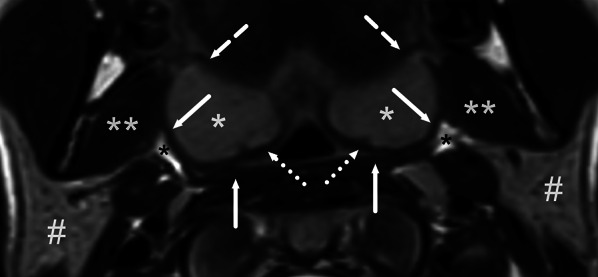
Normal MRI anatomy of the oropharynx. Axial T2-weighted image shows the palatine tonsils (white asterisk) bounded laterally by the superior constrictor muscle (arrow), anteriorly by the palatoglossus muscle (dashed arrow), and posteriorly by the palatopharyngeus muscle (dotted arrow). Posterolateral to the superior constrictor muscle and the buccopharyngeal fascia is the parapharyngeal space indicated by fat signal (black asterisk). The medial pterygoid muscle (double white asterisk) and the parotid gland (hash) can be seen in the masticator and parotid spaces, respectively
